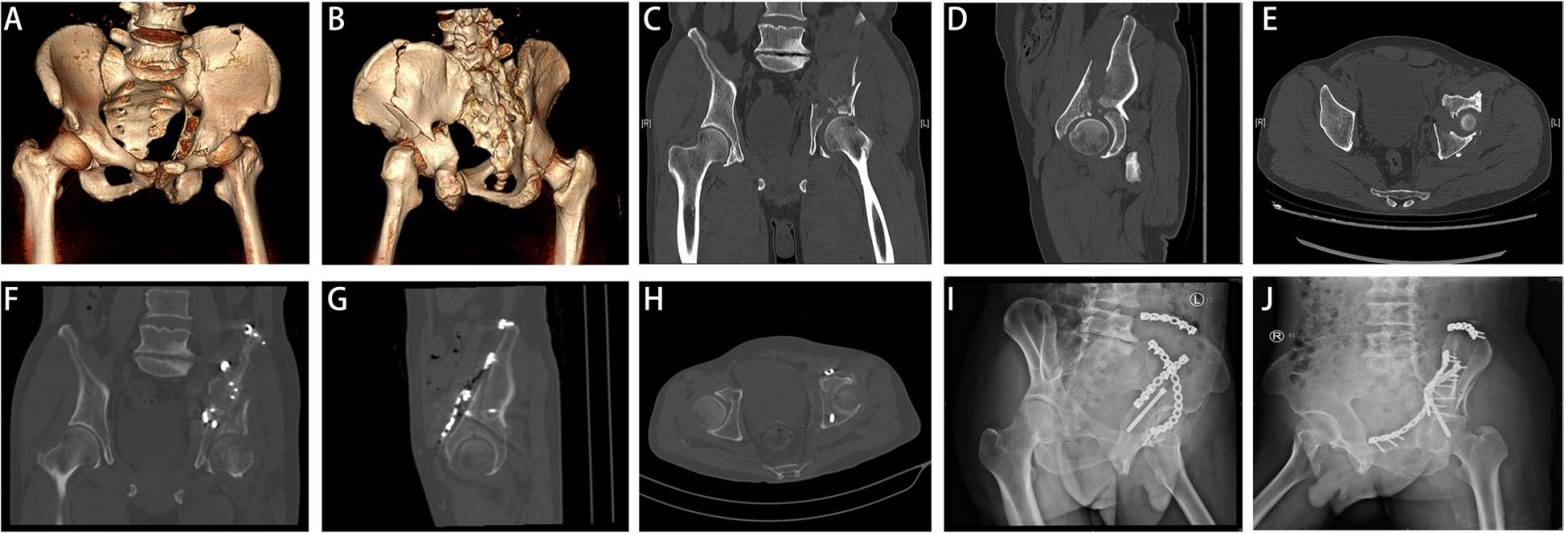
Preoperative and postoperative imaging evaluation. A, B Three-dimensional CT showed acetabular fractures involving both columns. C The coronal CT scan showed a dome impaction and a large “gap” of fragment preoperatively. D, E The sagittal and axial CT scan showed large “gap” and “step” of fragment preoperatively. F–H Postoperative CT scans showed the anatomical reduction and fixation with reconstruction plates. I, J Postoperative obturator oblique and iliac oblique views
